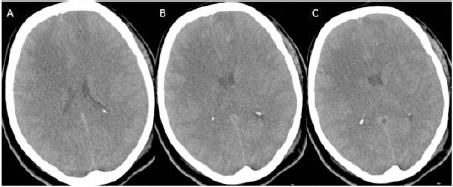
A, B, C: Cranial CT in axial sections following the biopsy procedure showing very little amount of bleeding*.
